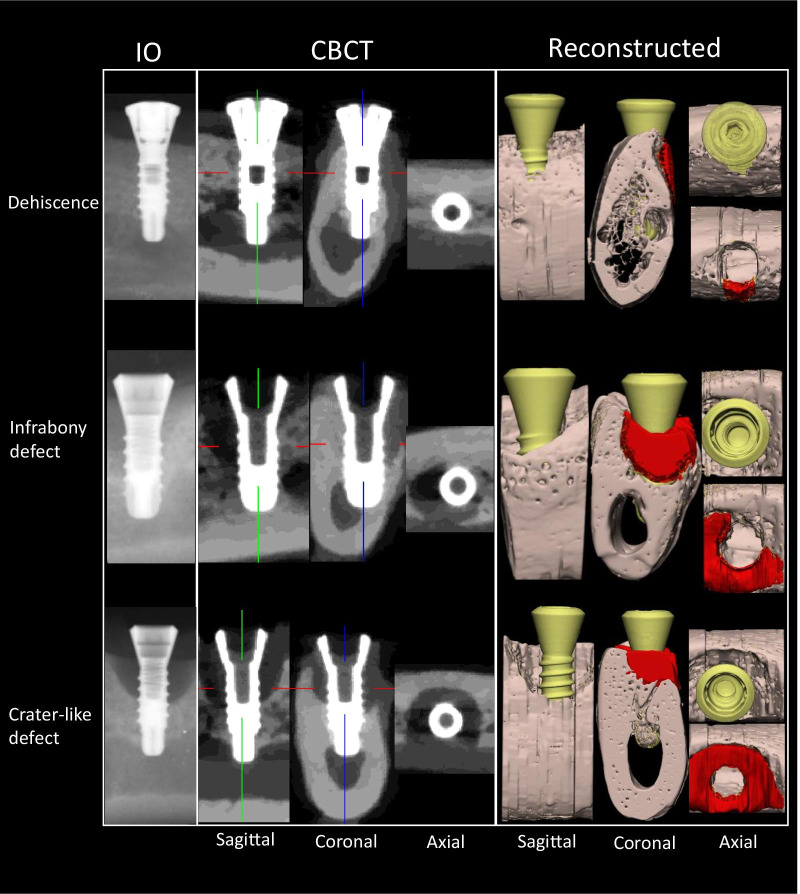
Shapes of peri-implant bone defects which were demonstrated in IO, CBCT and reconstructed imaging
